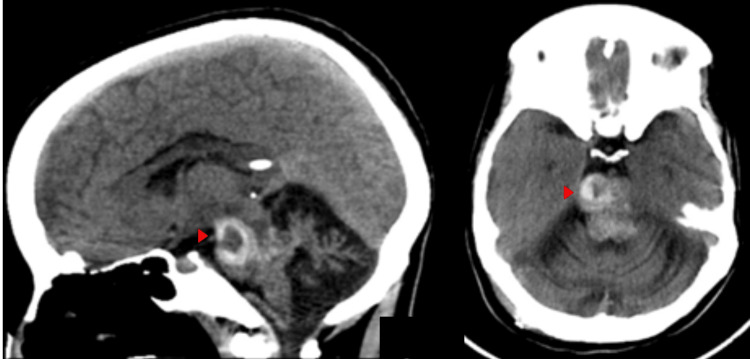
Urgent plain computed tomography imaging of the brain post shunt insertion Post shunt insertion sagittal (a) and axial (b) cut of non-contrast computed tomography scan showing lesional hyperdensity and regression in the size of the ventricles.
